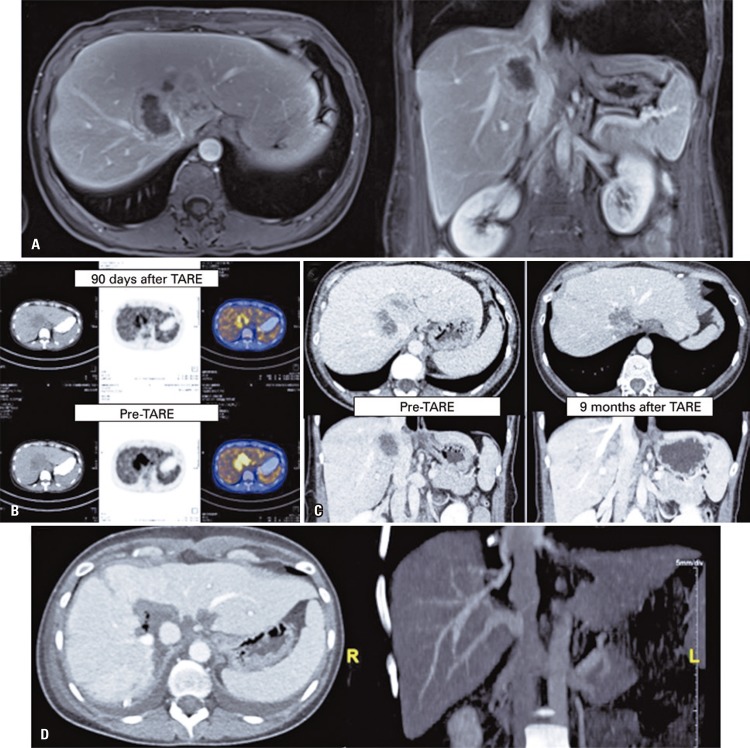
Patient with colangiocarciona who, initially, were candidate for resection. (A) Expansive injury 7.0 x 5.3cm, invading the cava vein and right and medium hepatic vein, which was suggestive of colangiomarcinoma. (B) Positron emission computed tomography conducted before and after treatment. (C) Computed tomography pre-radioembolization hepatic transarterial and follow-up (9 months after transarterial radioembolization of the liver), showing reduction of dimension of the injury and discreet contact with vascular structures. (D) Aspect of the liver in the computed tomography after resection of segments IV, VII and caudal resection
